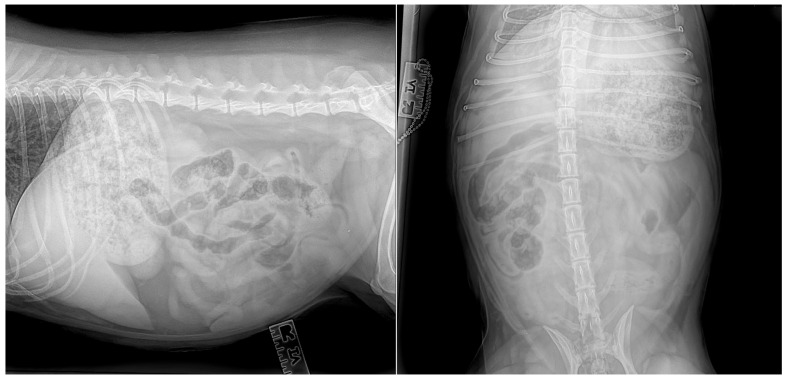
Abdominal radiographs revealed dilated intestines and mixed heterogeneous gas and soft tissue attenuating material within the stomach without evidence of a foreign body or obstructive pattern.
